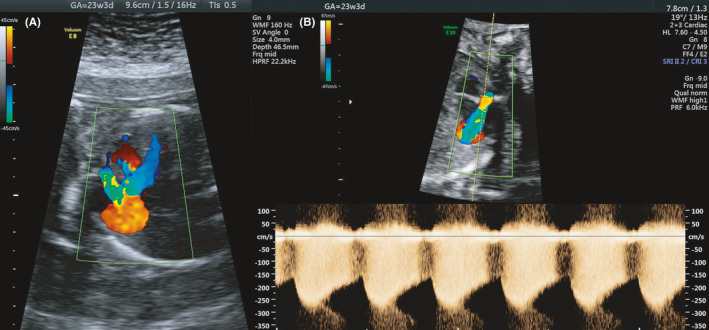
Color Doppler examination at 23 + 3 weeks of gestation showing the turbulent flow in the coronary artery fistula originating from the RCA draining to the right atrium (A). Continuous wave Doppler measuring maximum velocity of 3.2 m/s in the coronary fistula (B)
